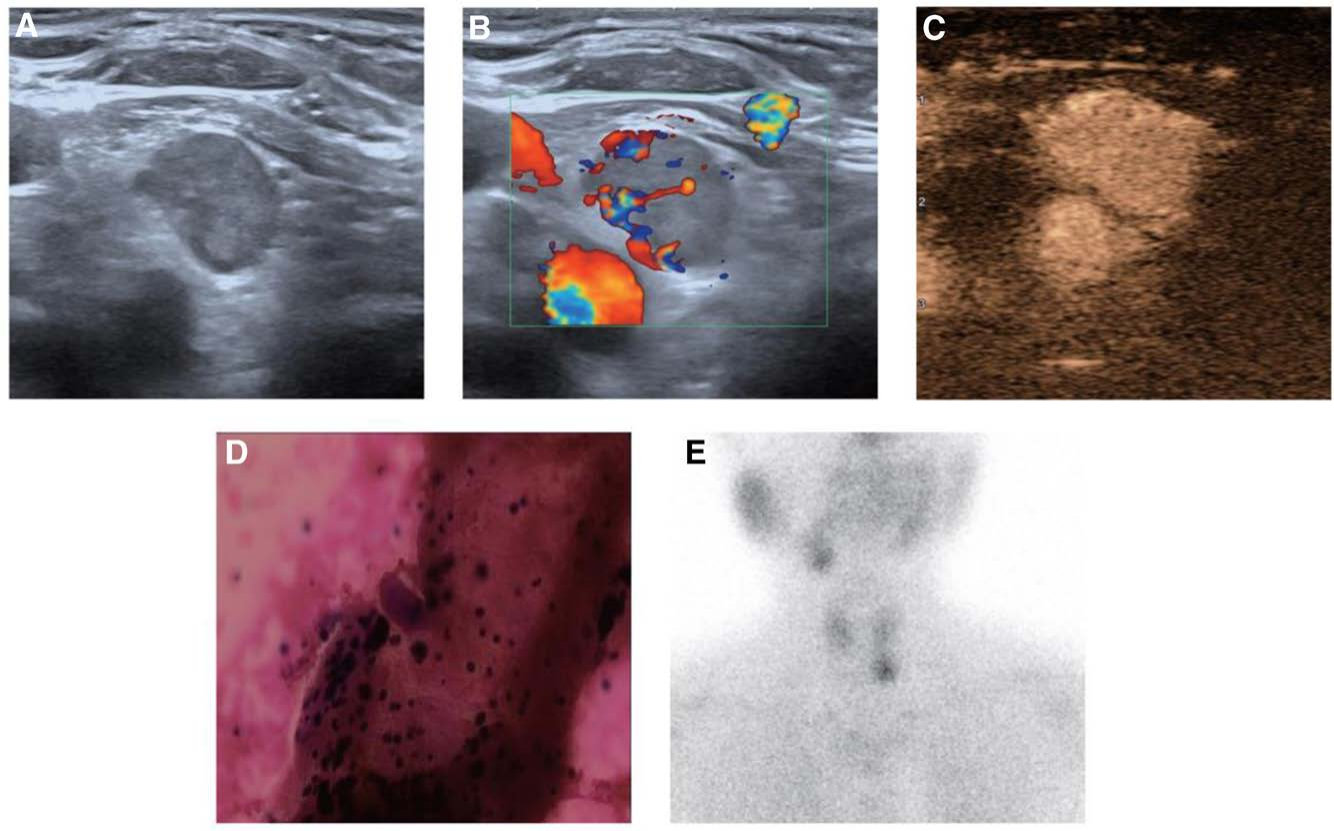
Preoperative examinations of the patient. (A) Conventional 2-dimensional ultrasound showed a low echo nodule on the dorsal side of the left inferior lobe of the thyroid, with clear boundary and irregular shape. (B) Color Doppler flow imaging (CDFI) showed that abundant blood flow signals could be detected inside and around the lesion. (C) Contrast-enhanced ultrasound (CEUS) showed that the lesion was uniformly enhanced during the arterial phase. (D) Pathology revealed that there were a small number of follicular epithelioid cells, and parathyroid cells were not excluded. (E) 99mTc-ses-tamibi SPECT showed localized nuclide concentration shadow at the lower pole of the left lobe of the thyroid gland. CEUS = contrast-enhanced ultrasound, CDFI = color Doppler flow imaging, SPECT = single photon emission computed tomography.
